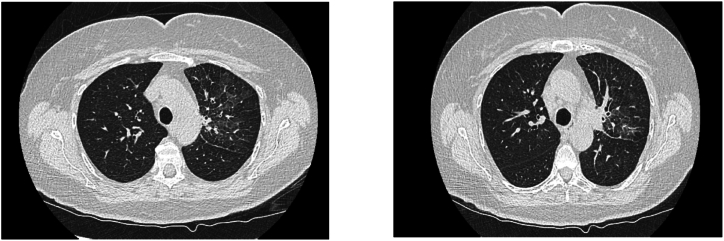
CT images of the chest after 3 weeks while the patient remained on corticosteroids and off alectinib, the previously ground-glass opacities were resolved.
